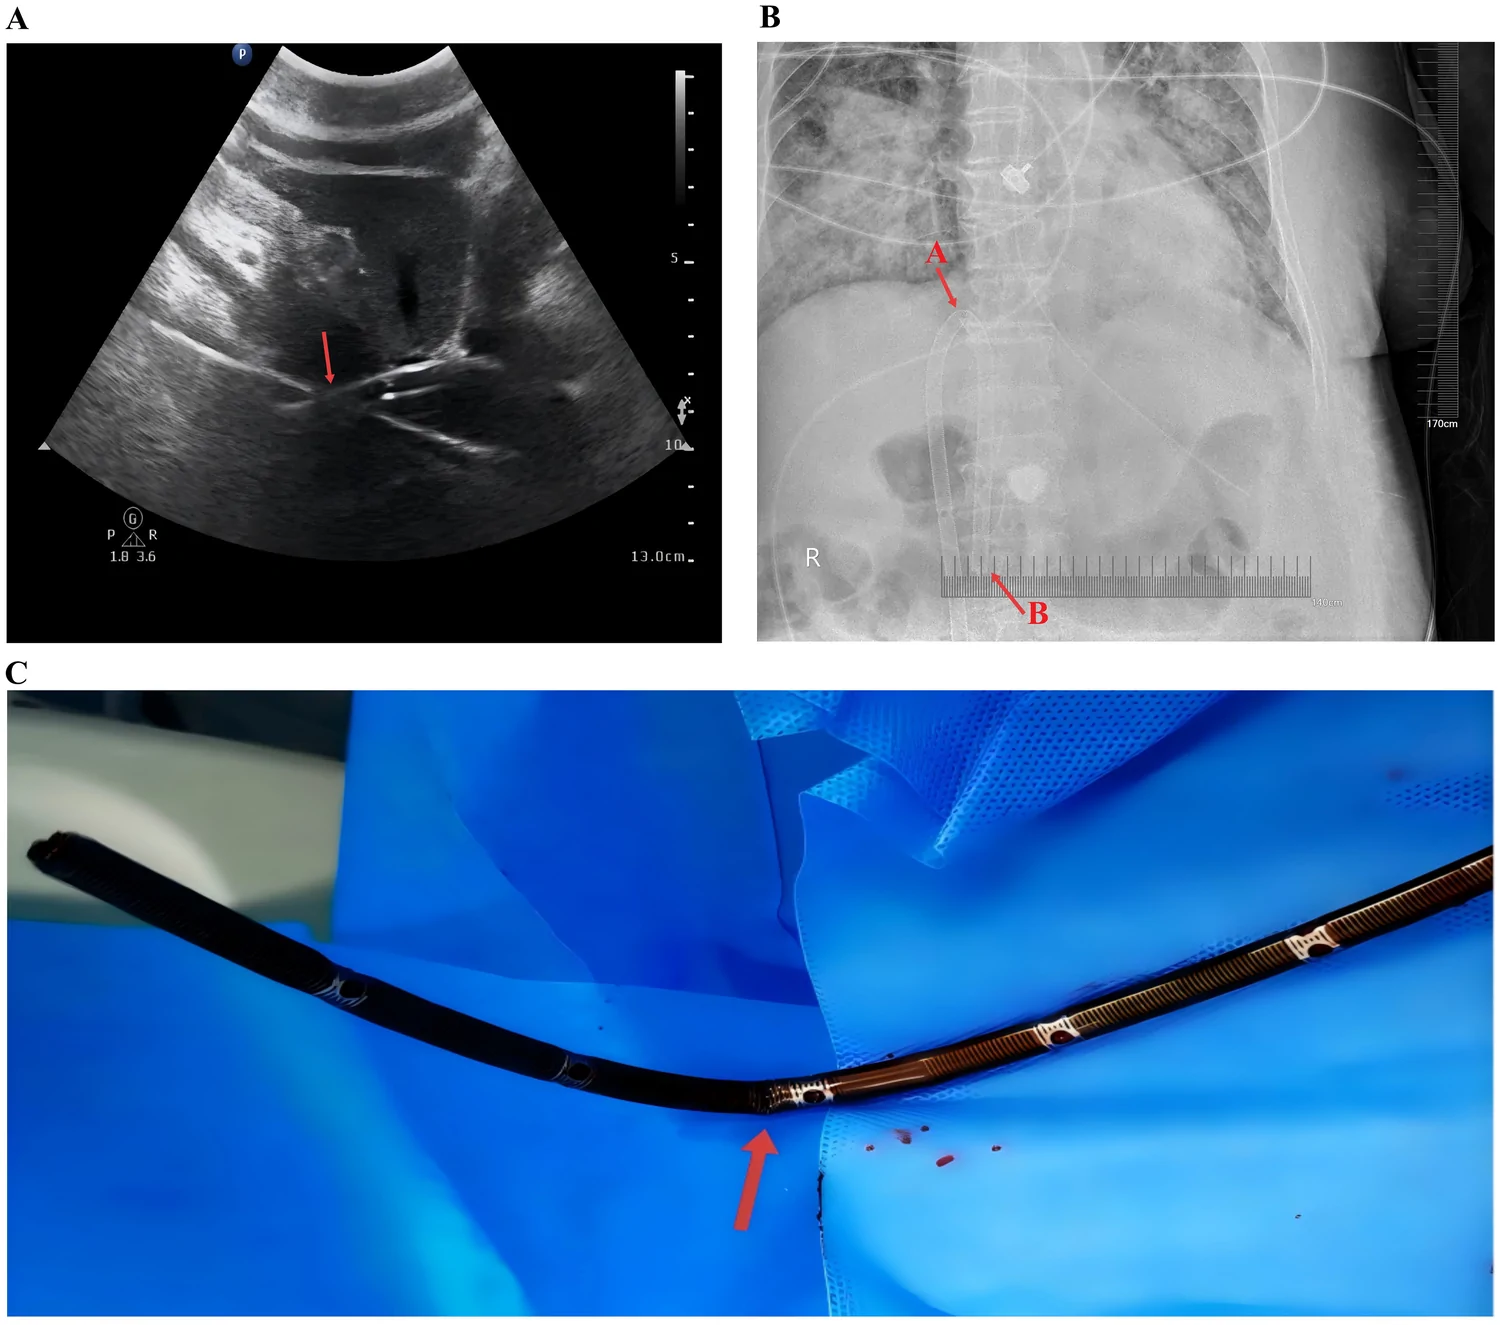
Imaging findings and post-removal appearance of the fold-back kink of the VA-ECMO venous drainage cannula. (A) Bedside ultrasonography showed that the ECMO venous drainage cannula remained within the vascular lumen, with an obvious focal bending configuration. The arrow indicates the kinked segment of the cannula. (B) Bedside radiography showed a marked fold-back kink of the ECMO venous drainage cannula inserted through the femoral vein along the course of the IVC. Arrow A indicates the site of cannula bending/fold-back deformation, and arrow B indicates the position of the cannula tip. (C) Appearance of the venous drainage cannula after VA-ECMO decannulation. The arrow indicates persistent bending of the cannula. The cannula remained structurally intact, with no fracture or visible damage.
